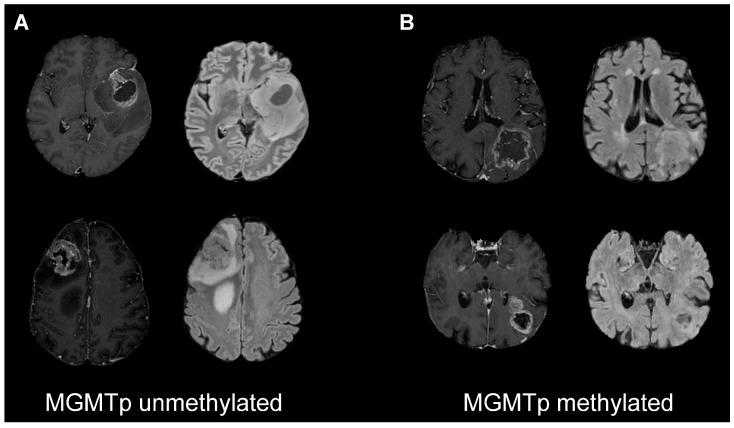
Post-contrast T1-weighted and FLAIR images of four representative patients with MGMTp-unmethylated (A) and -methylated (B) tumors. The selected patients differed in the combinations of the three most important features for classification: high values of GLRLM long-run high gray-level emphasis ((A) MGMTp-unmethylated tumors), high values of GLSZM gray-level non-uniformity normalized ((A) MGMTp-unmethylated tumors), and high values of GLSZM low gray-level zone emphasis ((B) MGMTp-methylated tumors). Abbreviations: MGMTp = O6-methylguanine-DNA methyltransferase promoter; FLAIR = fluid-attenuated inversion recovery; GLRLM = gray-level run length matrix; GLSZM = gray-level size zone matrix.
